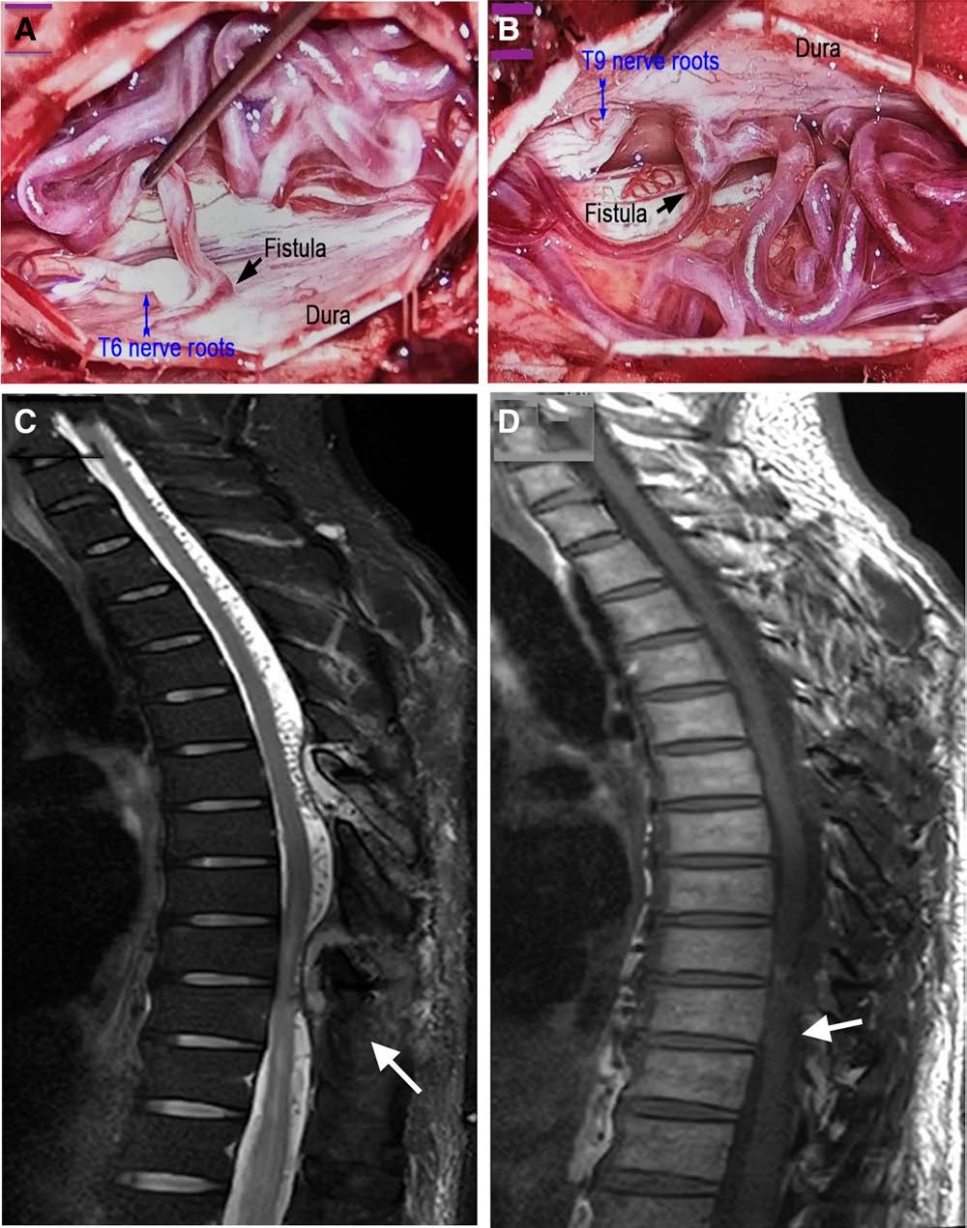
Intraoperative images and postoperative spinal magnetic resonance imaging (MRI). (A, B) Fistulas (black arrows) and draining veins around the T6 and T9 nerve roots that were successfully excised. (C) A T2 weighted image showing disappearance of spinal cord edema and superficial tortuous vascular shadows on MRI after surgery (white arrow). (D) A T2 weighted image showing disappearance of spinal cord edema and superficial tortuous vascular shadows on MRI after surgery (white arrow).
